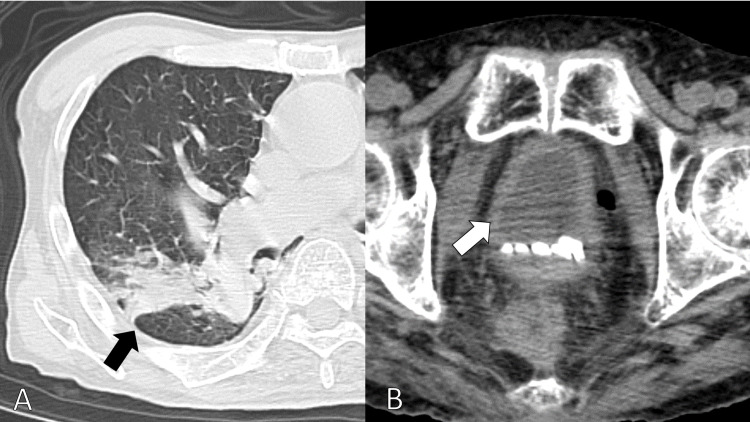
CT scan from the neck to the pelvis showing infiltration of the right lung (A). And no high-density areas in the bladder indicative of hemorrhage (B).
